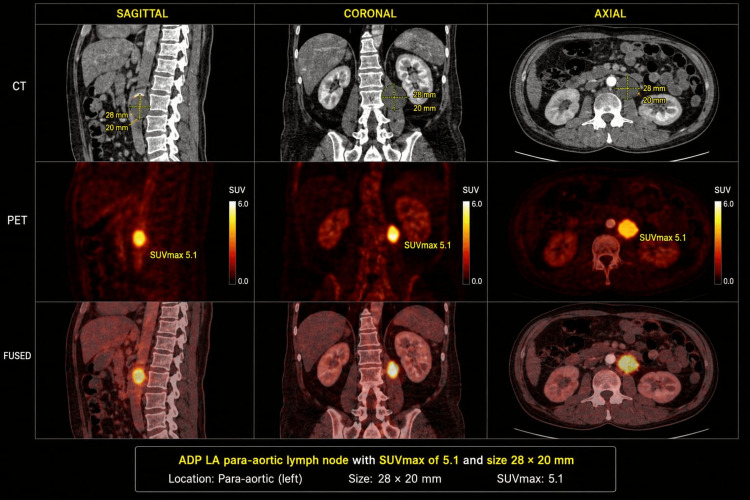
Diagnostic PET-CT of Patient 2 showing isolated para-aortic nodal recurrence. A solitary hypermetabolic para-aortic lymph node was identified without evidence of additional regional or distant disease. PET-CT: positron emission tomography-computed tomography; SUVmax: maximum standardized uptake value
